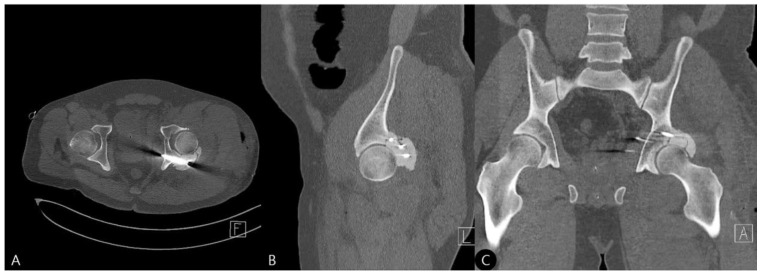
Postoperative computed tomography (CT) scans. (A) Axial, (B) sagittal, and (C) coronal views confirming anatomical reduction in the femoral head, reconstruction of the posterior wall defect, and the relationship between the screw framework and PMMA cement. “F” denotes the feet side, “L” denotes the left side, and “A” denotes the anterior side.
